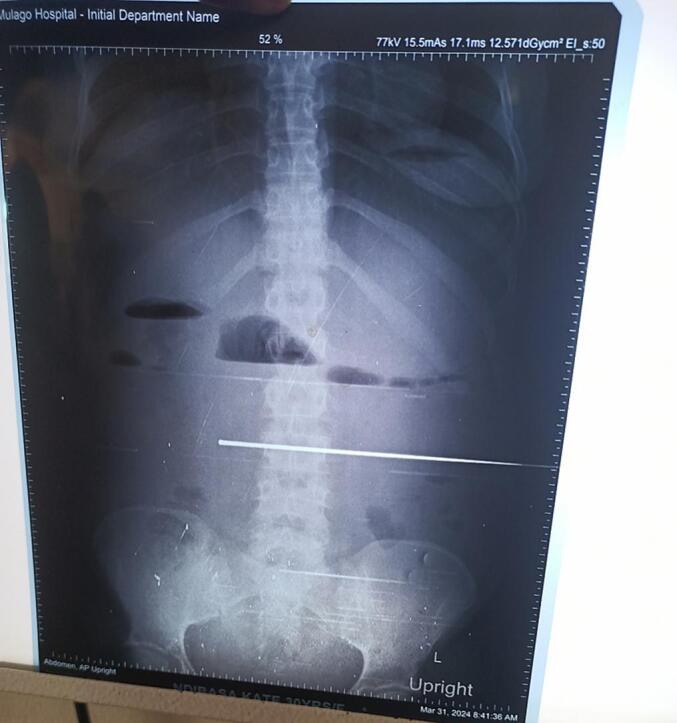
Erect Abdominal X-ray showing five air fluid levels.
